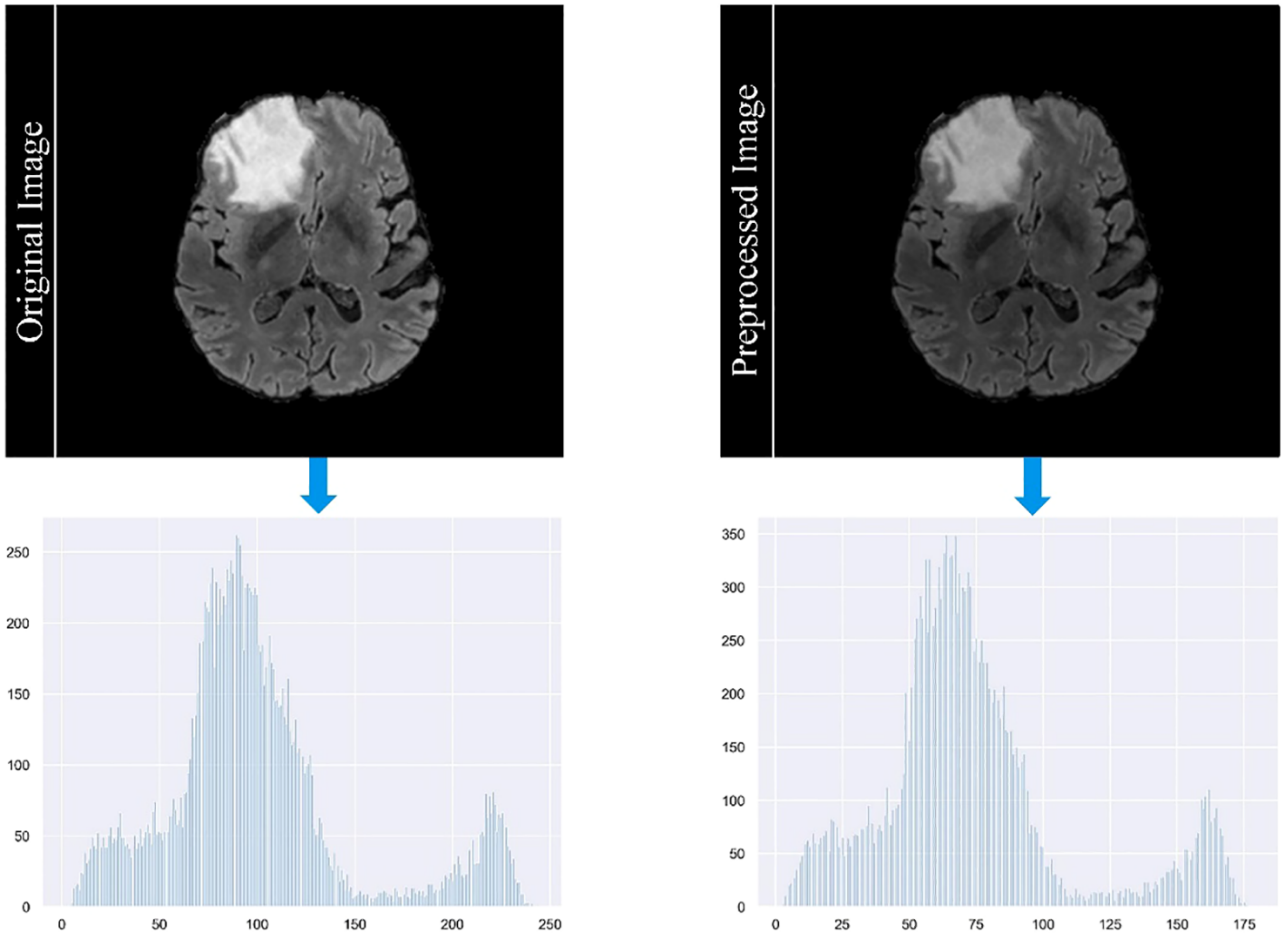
Comparison of MRI images before and after the standardization process.
